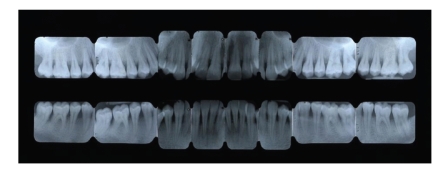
X-ray examination at baseline.
